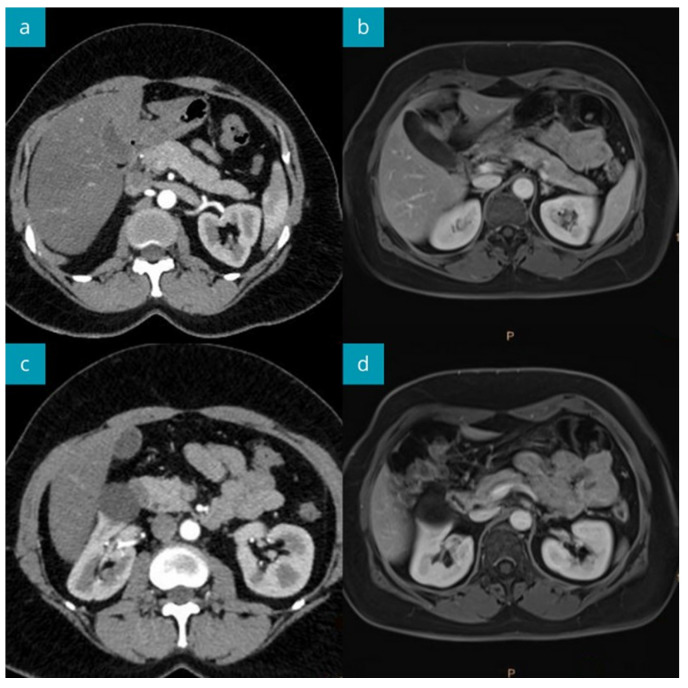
The abdominal CT imaging during the primary diagnostic work-up (a,c), and MRI after 15 months of follow-up (b,d). The head (mildly enlarged), body, and tail of the pancreas show normal structure. Normal pancreas image in MRI performed 15 months later.
